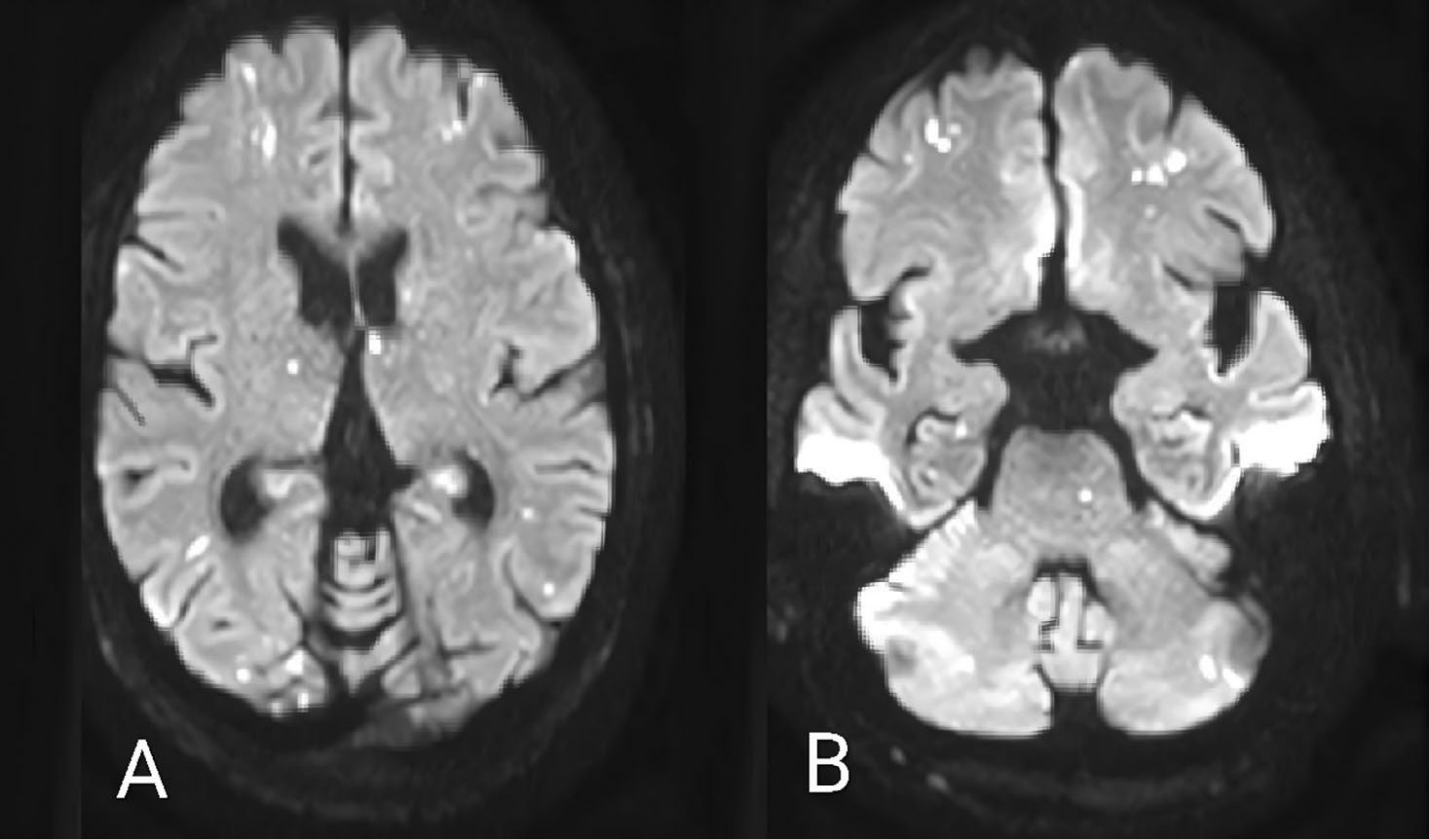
Brain magnetic resonance imaging (A and B) revealed multiple T2‐hyperintensities with restriction, indicative of embolic stroke infarcts affecting cortex, subcortical white matter, caudate nucleus, pons and cerebellum.
